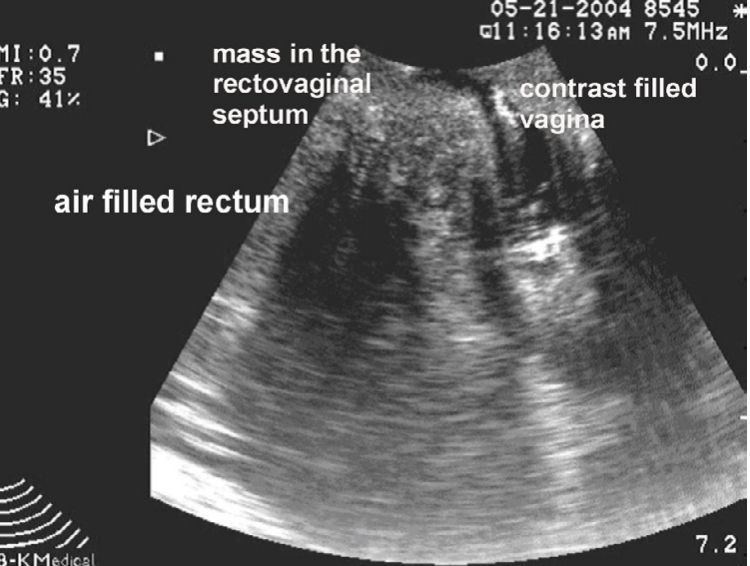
Sagittal transperineal sonography showing the recurrent mass in the same patient occupying the rectovaginal septum. The vagina has been filled with acoustic contrast.
